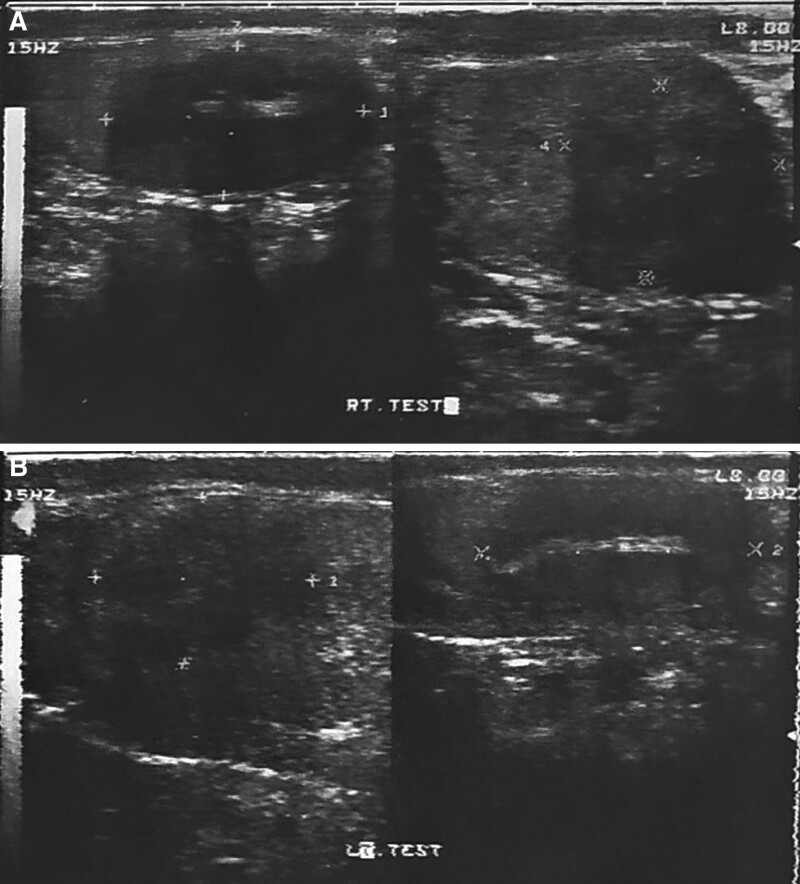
Gray-scale ultrasound images of the right (A) and left testes (B). Both testes are mostly occupied by hypoechoic lesions with clear irregular margins and linear increased echogenicity in the central area.
